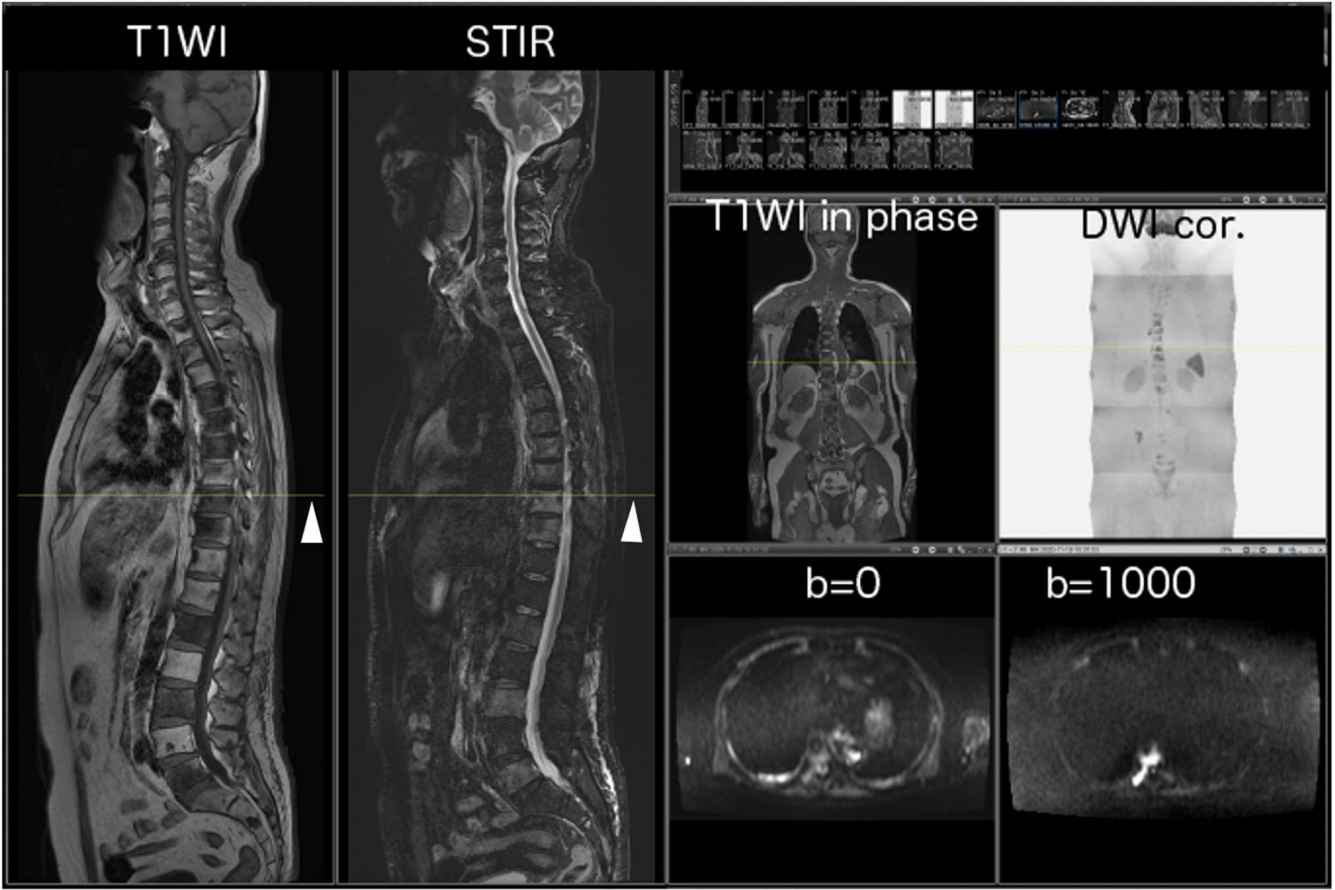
Imaging layout. This image was captured using WB-MRI by the radiologists and comprised two monitors. On the left side of the monitor, the sagittal T1W images and STIR images are displayed by longitudinal two partings, and on the right side of the monitor, the coronal in-phase T1W images, coronal reconstructed DW images, axial *b* = 0, and axial *b* = 1000 images were displayed by four partings. For all image planes, reference lines were used to detect the precise level of the regions (white arrowheads)
